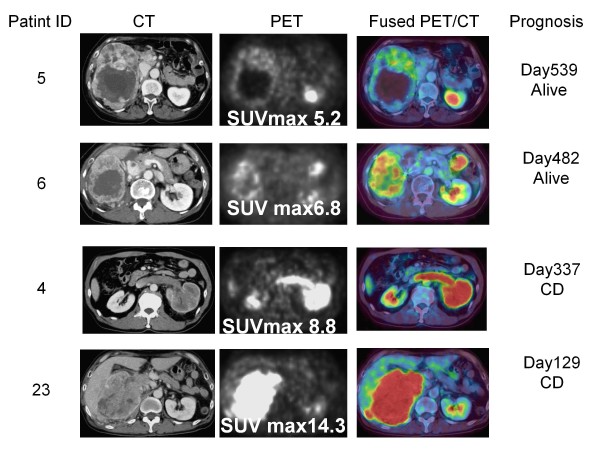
Four Cases with advanced RCC which original sites showed the highest value of SUV among all RCC sites and their prognosis. The patients with advanced RCCs having high values of SUV max demonstrated poor clinical courses. SUVmax, maximum standardized uptake value; CT, computed tomography; PET, positron emission tomography; Fused PET/CT, fusion of PET and CT.
